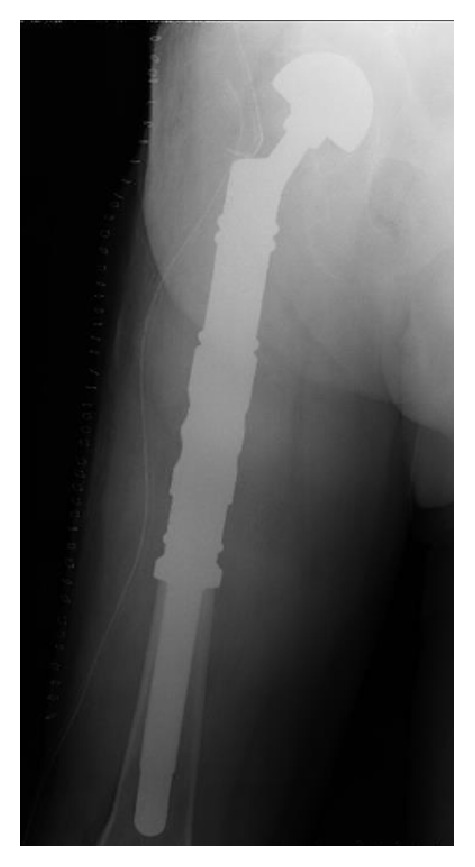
Postoperative X-ray after proximal femur resection and reconstruction with silver-coated modular prosthesis (MUTARS Implantcast Ltd., Buxtehude, Germany).
